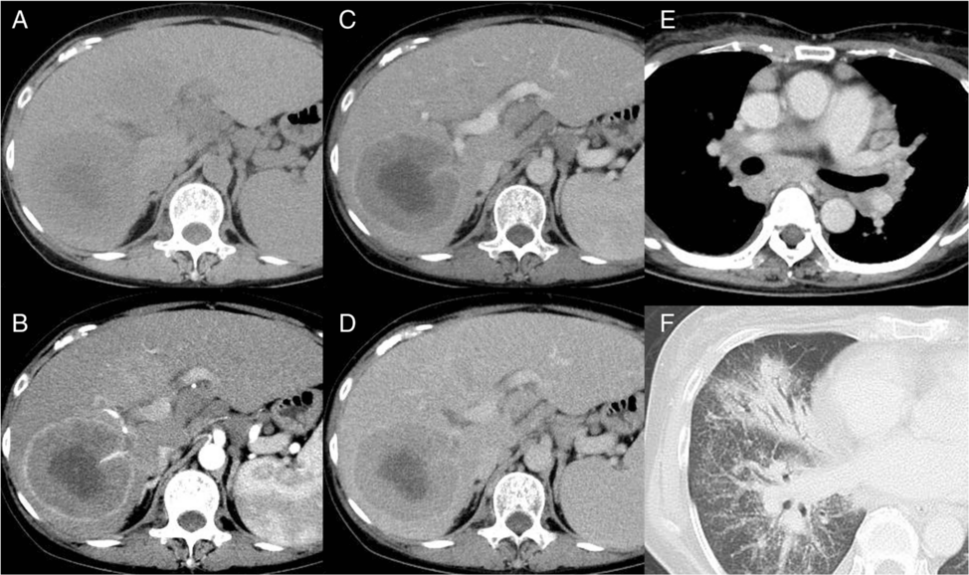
Abdominal dynamic CT images in a 54-years old female with sarcoidosis–lymphoma syndrome. a-d Plain CT image shows two well-circumscribed, peripherally-enhancing, low-density masses in the anterior segment (S5; 80 × 78 × 88 mm) and inferior segment (S7; 62 × 88 × 82 mm) of the right lobe of the liver. In the arterial phase, a hepatic artery is seen penetrating inside the tumor. *CT* computed tomography. Chest CT images in a 54-years old female with sarcoidosis-lymphoma syndrome. e Contrast enhancement shows mediastinal and hilar lymphadenopathies. f On lung window, there is irregular thickening of the bronchovascular bundles and central peribronchial fibro-infiltration lesions in the right middle and lower lobes
